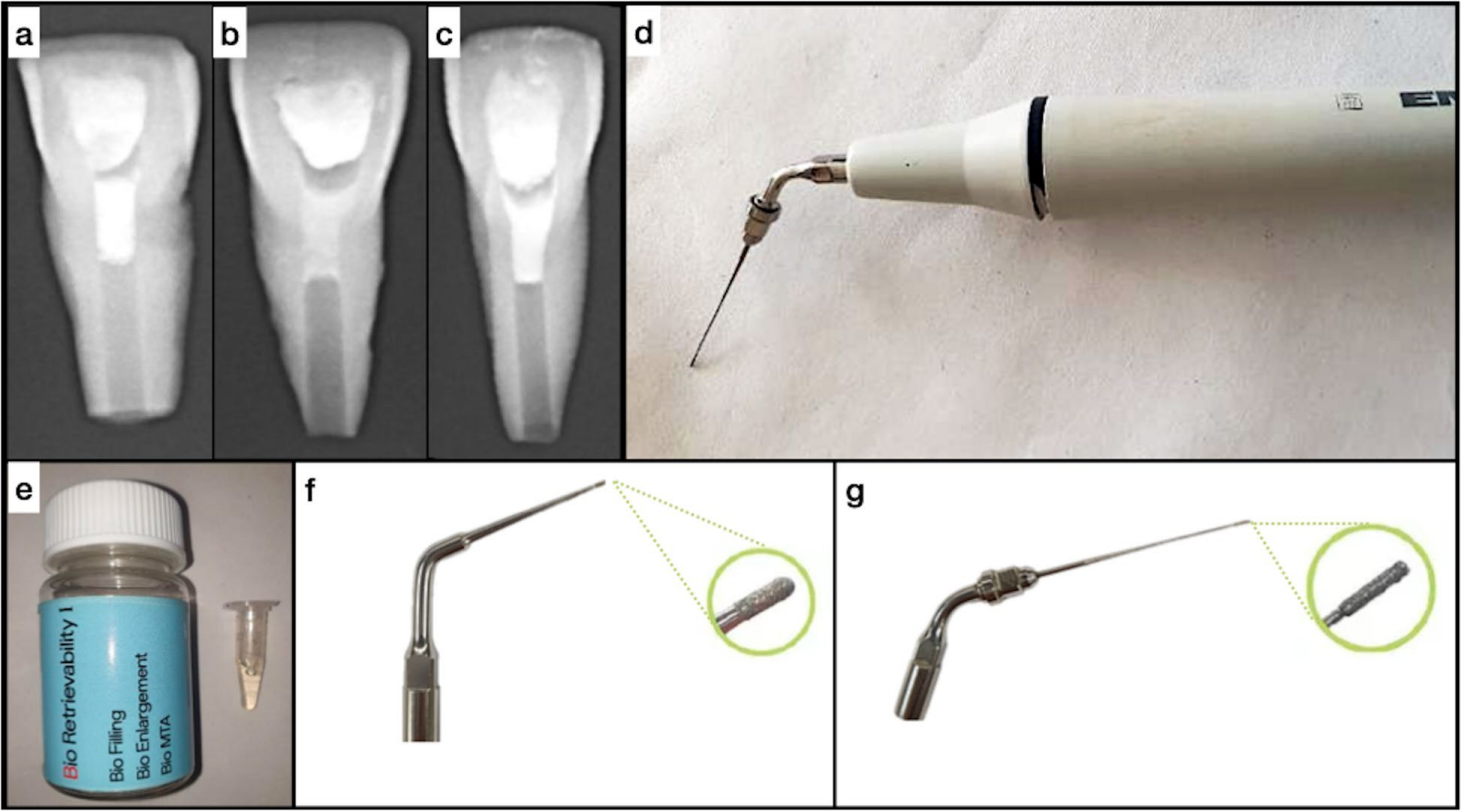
X-ray image data was acquired due to confirm the proper placement of (a) ProRoot MTA, (b) BioDentine, and (c) RetroMTA. d shows the Bust-05 tip and the attachment to the ultrasonic device. e shows the glycolic acid liquid used for softening capping material. f shows the magnification of the diamond-coated large ultrasonic tip (Bust-05). g shows the magnification of the diamond-coated fine ultrasonic tip (Bust-03)
